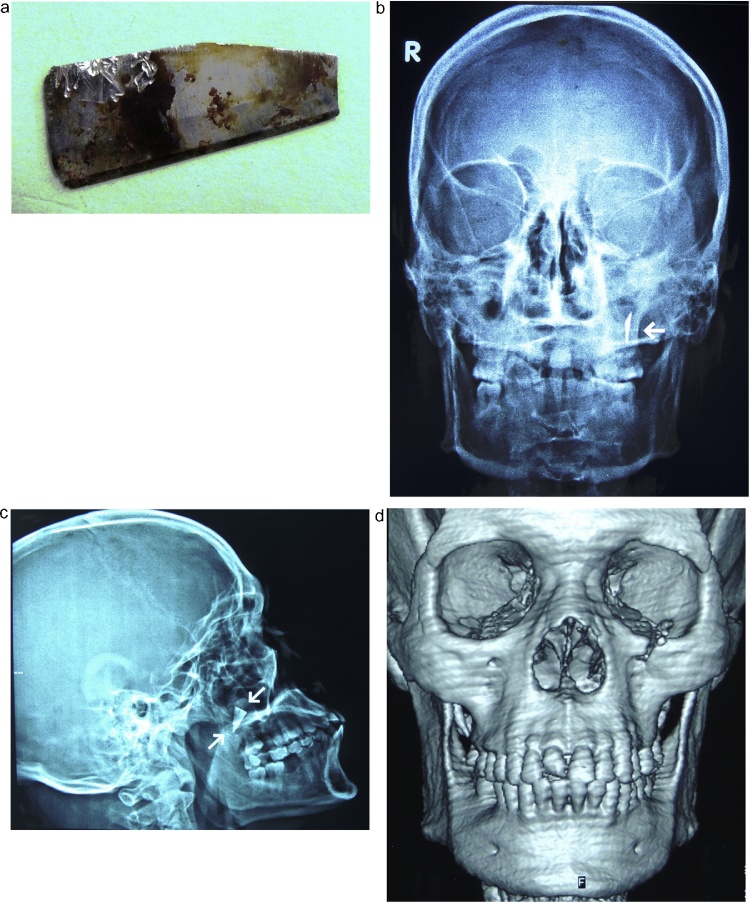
Showing the immediate postoperative results (a) the extracted knife blade (the foreign body) (b, c) posteroanterior and lateral skull views respectively showing the retained tip of the foreign body. (d) postoperative 3D computed tomography showing the resultant defect in the inferior orbital rim.
